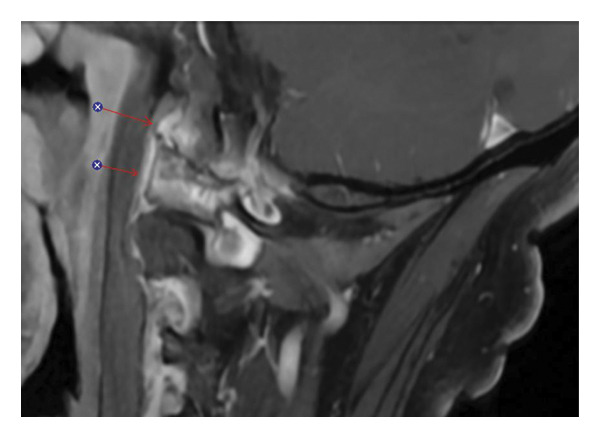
Sagittal fat‐suppressed postcontrast T1‐weighted MRI demonstrating enhancement of the periodontoid soft tissues and left atlanto‐occipital region corresponding to the areas of edema seen on the STIR sequence in Figure 1 (arrows).
